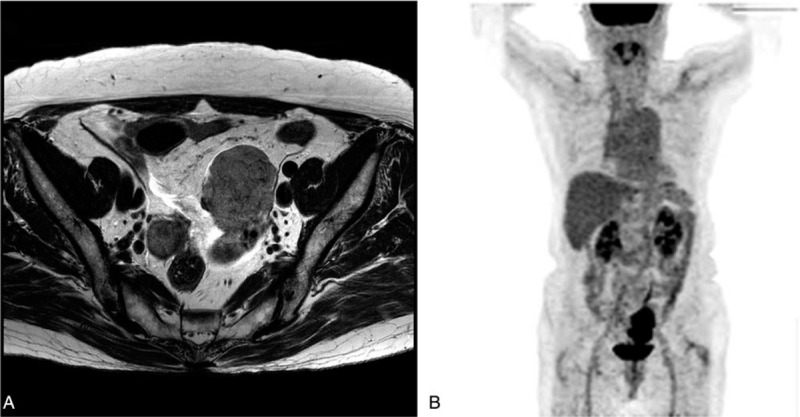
At diagnosis, (A) pelvic magnetic resonance images of a predominantly solid mass, measuring 5.5 cm × 4.6 cm, with a lobulated contour in the left ovary. (B) ^18^Fluoro-2-deoxy-D-glucose positron emission tomography/computed tomography images of a 5.8 cm × 4.2 cm FDG avid mass in the left ovary.
